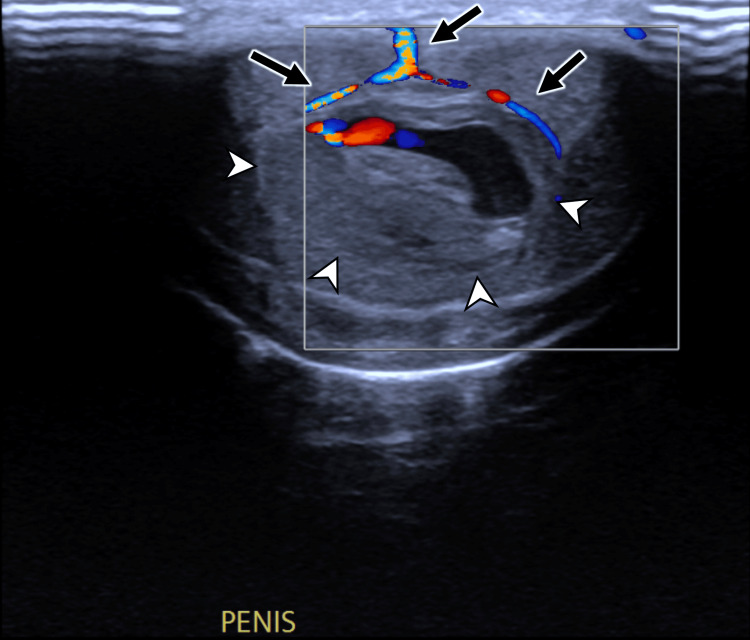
Colour Doppler ultrasound of the penis in transverse section view Note how branches of the dorsal penile vein (black arrows) are splayed by the central haematoma (white arrowheads).
